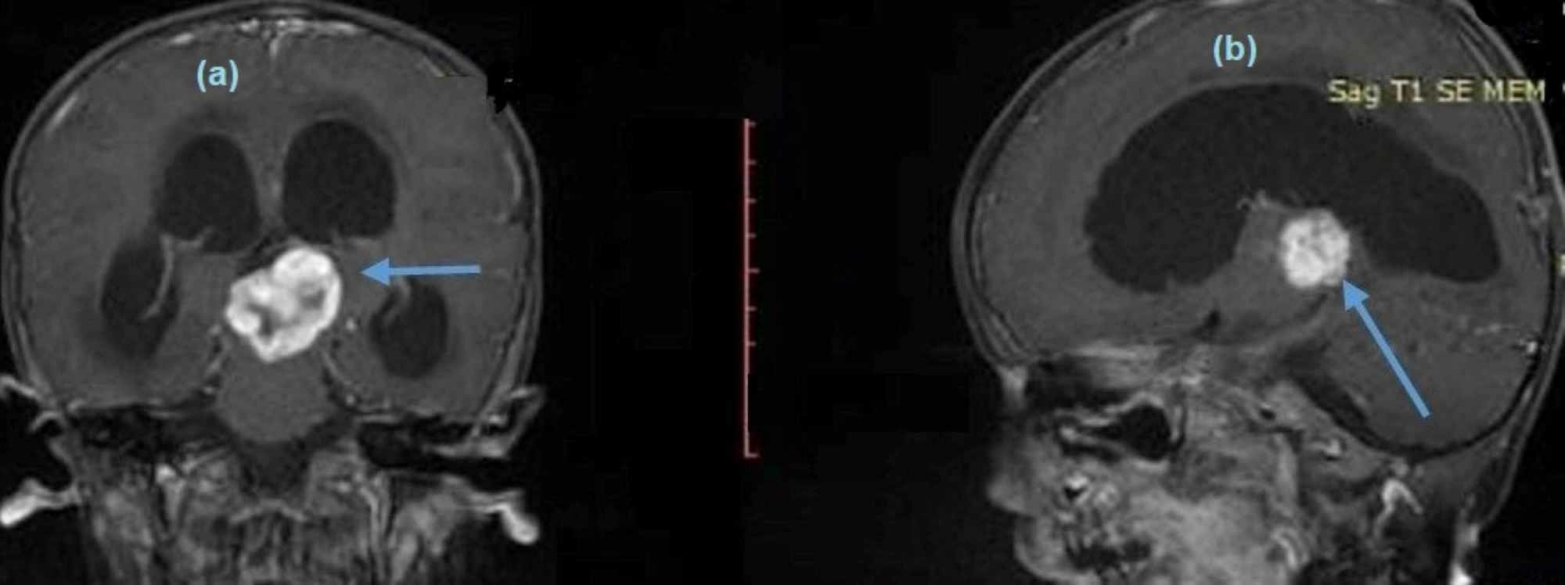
Magnetic resonance imaging (MRI) of the brain showing a homogenous hyperdense and enhancing mass in the pineal region in coronal (a) and sagittal (b) planes.
